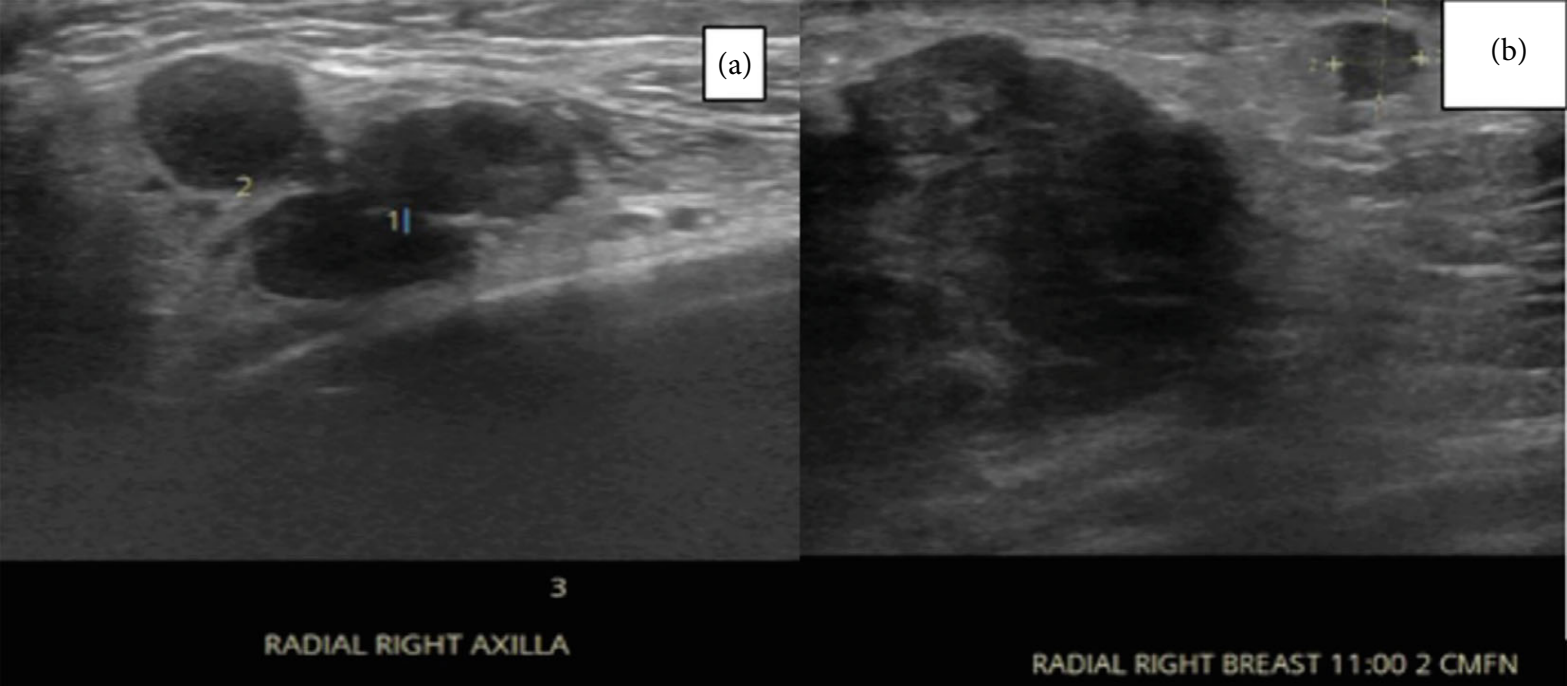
Ultrasound of the right breast and axilla. (a) Targeted breast ultrasound showing a hypoechoic irregular mass with posterior acoustic shadowing, suggestive of malignancy. (b) Axillary ultrasound revealing multiple enlarged, rounded lymph nodes with cortical thickening and loss of fatty hilum, compatible with nodal metastases.
